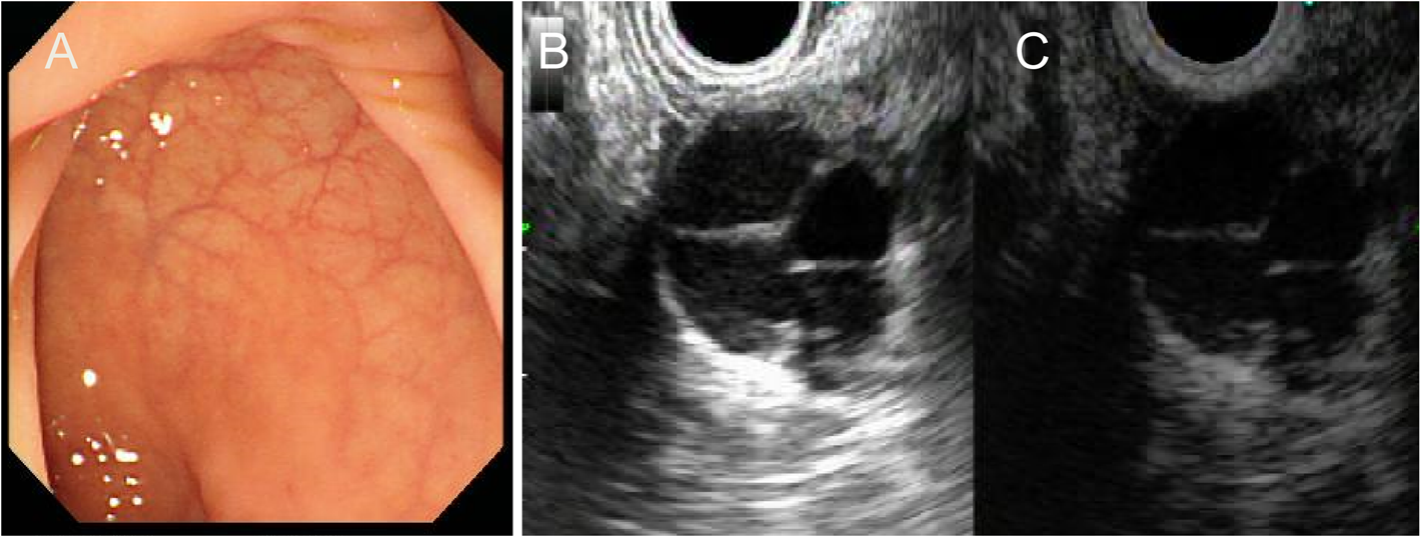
Colonoscopy and endoscopic ultrasound. a No rectal submucosal tumor is seen on colonoscopy. b The endoscopic ultrasonography (EUS) shows a hypo- and anechoic multilocular mass measuring 20 mm in diameter with clear boundaries. c Only the cyst wall is enhanced on contrast-enhanced ultrasonography (CE-US)
